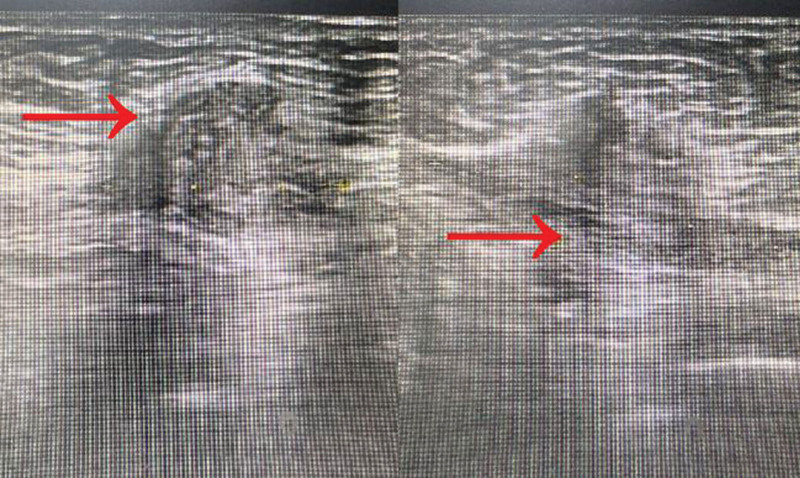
Preoperative ultrasound of abdominal wall masses showed that hypoechoic mass was found in the soft tissue of the abdominal wall, ranging from 18 mm × 41 mm × 27 mm, with irregular shape, clear boundary, and uneven internal echo.
